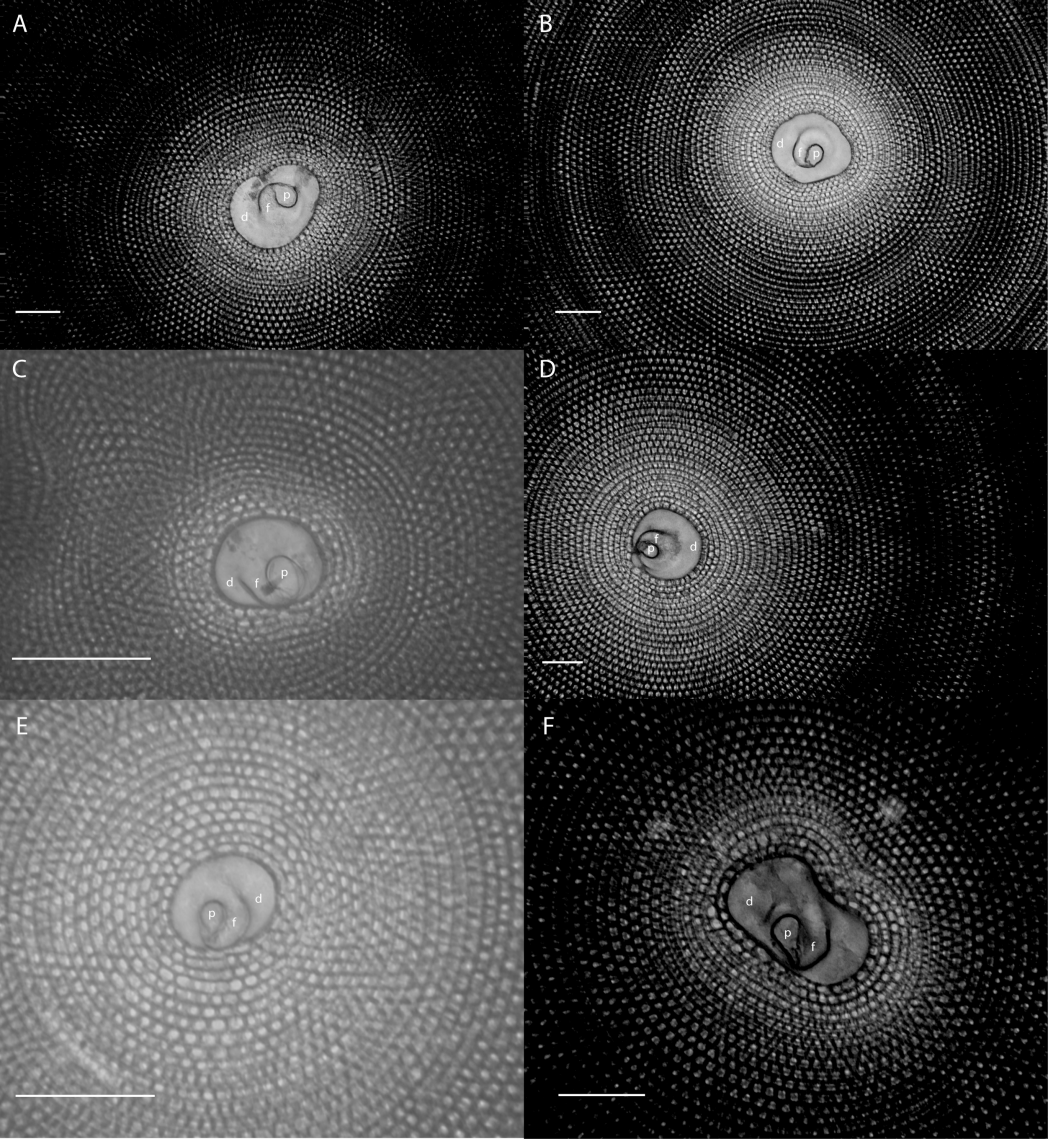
X-ray projections of the center of the shell of *Marginopora* spp. A) *M*. *rossi* (RGM.1352064) with the deuteroconch (d) completely enveloping the protoconch (p) and flexostyle (f), B) *M*. *charlottensis* nov. spec. (RGM.1352050); C) *M*. *vertebralis* (RGM.1352105); D) *M*. *orpheusensis* nov. spec. (RGM.1352059); E) *M*. *santoensis* nov. spec. (RGM.1352122); F) *M*. *charlottensis* nov. spec. (RGM.1352055). Especially in the populations with larger deuteroconchs, irregularly shaped deuteroconchs are frequent, resulting in more variance in the deuteroconch diameters. Scale bar 500μm.
